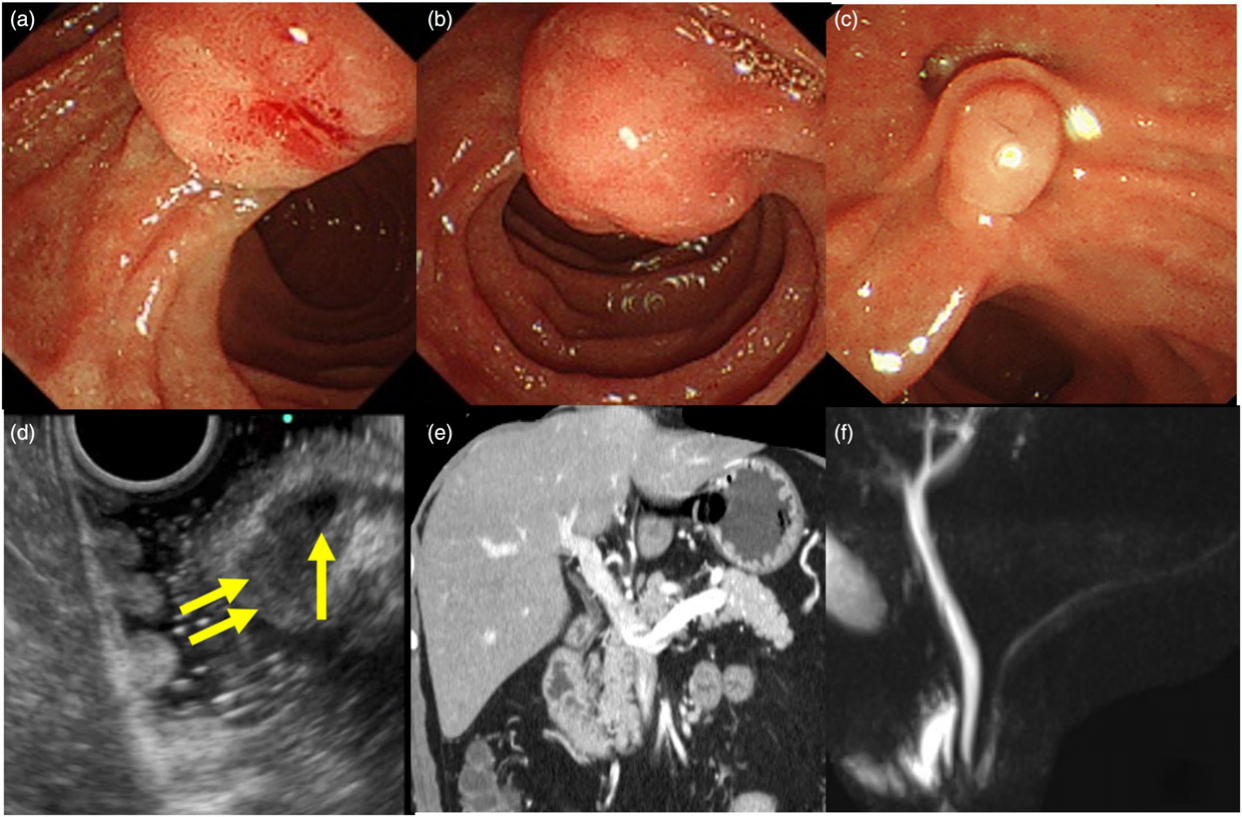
Abdominal examination images. (a) Upper gastrointestinal endoscopy showing an enlarged view to about 10 mm minor papilla. (b) Duodenoscopy showing a submucosal epithelial lesion in the minor papilla. (c) Duodenoscopy showing the major papilla, but no significant changes were noted. (d) Endoscopic ultrasonography showing an 8 mm hypoechoic neoplasm within the submucosal layer. There is dilation of the duct of Santorini, without invasion of the muscularis propria or intraductal extension to the pancreatic duct (arrow) showed dilation of the duct of Santorini and (double arrow) showed a hypoechoic neoplasm. (e) Abdominal computed tomography showing no distant metastasis. (f) Magnetic resonance cholangiopancreatography does not show pancreatic divisum.
